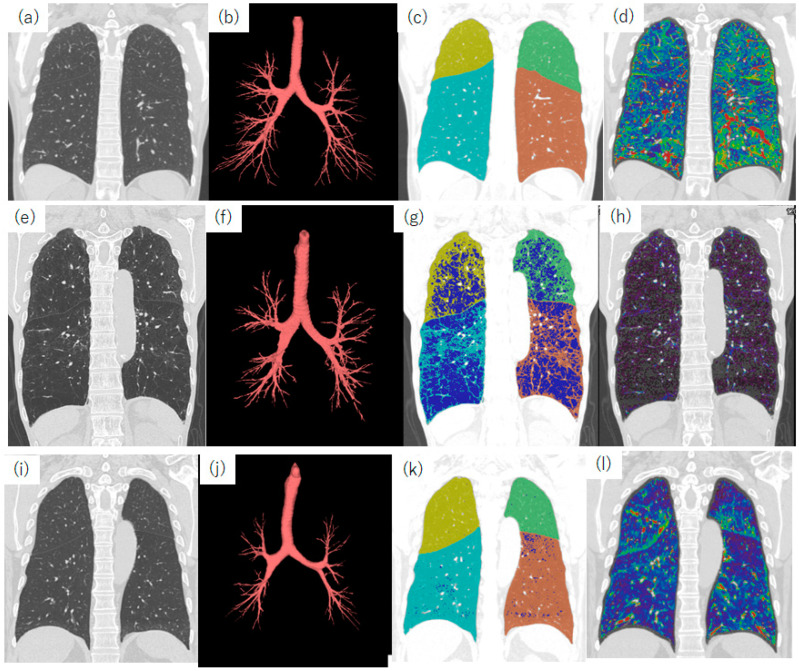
Air trapping in registered images between inspiratory and expiratory CT. (a–d) Images of a 55-year-old female never-smoker with normal respiratory function. (e–h) Images of an 81-year-old male patient with COPD who received home oxygen therapy. His lung function measurements showed an FEV1/FVC ratio of 26.6% and a %FEV1 of 42.3%. (i–l) Images of a 73-year-old male patient with COPD. His lung function measurements showed an FEV1/FVC ratio of 64.4% and a %FEV1 of 75.1%. (a,e,i) Coronal reconstructed standard-dose CT images. (b,f,j) Segmented bronchial trees. (c,g,k) %LAA results. Blue color indicates pixels with attenuation values below −950 HU. (d,h,l) Registered images between inspiratory and expiratory CT scans. The color indicates the difference in attenuation values between inspiratory and expiratory CT images. Red represents a large difference, blue indicates a small difference, and violet represents almost no difference. In the patient in the bottom row, the %LAA is 2.5%; however, marked air trapping is observed. The peripheral bronchial lumen is not segmented. These results indicate the airway subtype of COPD. Abbreviations: COPD, chronic obstructive pulmonary disease; FEV1, forced expiratory volume in one second; FVC, forced vital capacity; HU, Hounsfield unit; and LAA, low-attenuation area.
